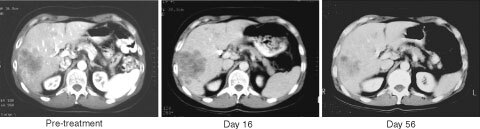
Abdominal CT-scans from a patient with liver metastases from colorectal cancer who received infusions of ONYX-015 into the hepatic artery in combination with 5-FU and Leucovorin (i.v. bolus injection) as part of a phase-II clinical study (Table 1
